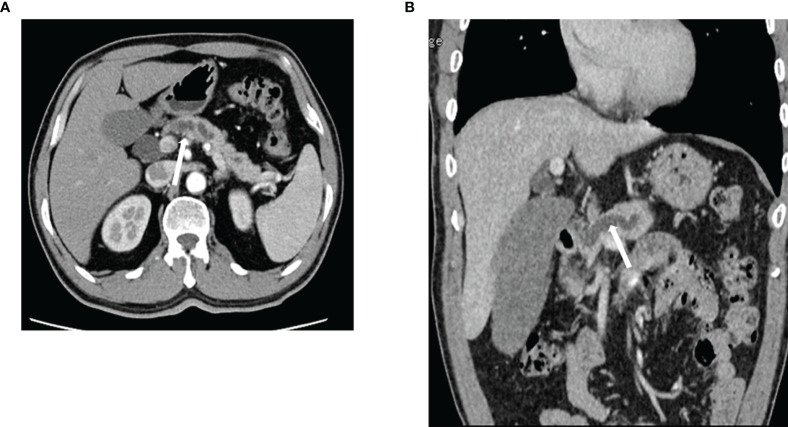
Expansion of the pancreatic duct. ACC in a 63-year-old man (A, B) Contrast-enhanced computed tomography reveals ductal dilatation. ACC, acinar cell carcinoma.
